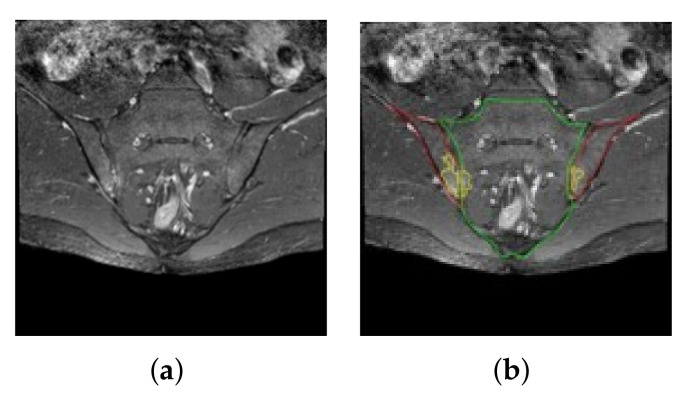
Example MR images of the SIJ region. (a) MR images including all areas surrounding the sacral and iliac bones; (b) annotated MR images of the sacral (green) and iliac (red) bones as well as bone marrow edema (yellow).
